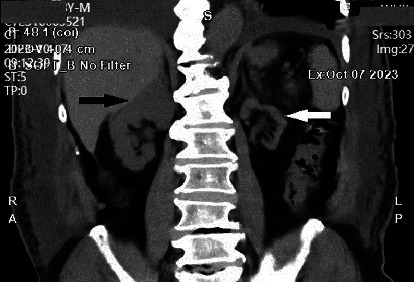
CT image in 2023 shows left renal atrophy not recovered (white arrow). Right kidney compensatory hypertrophy (black arrow).
